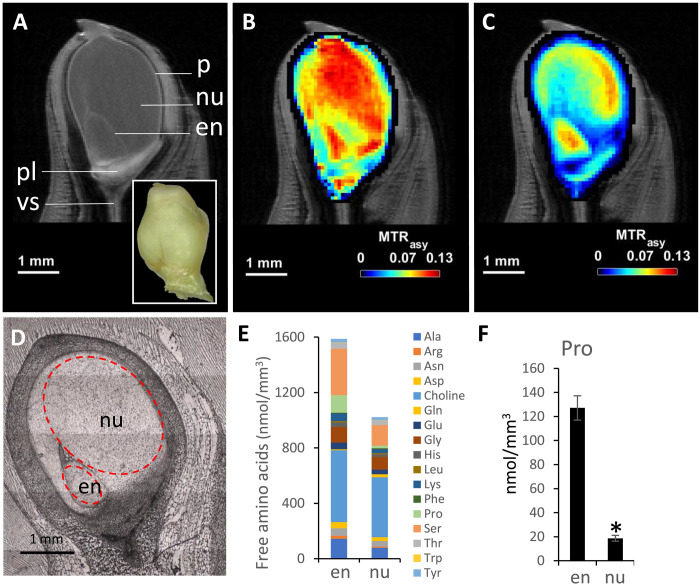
Noninvasive imaging of sugar and amino acid distribution by CEST and metabolite quantification by LC-MS in the early maize kernel. (A) MRI reference image visualizing the internal structure of the maize kernel (6 DAP). Photographic image of intact kernel on insert. (B and C) CEST image of sugars (B) and amino acids (C) in the living maize kernel. Image resolution, 40 μm. (D) Cryosection of the kernel used for microdissection of the tissues for detection of free amino acids; red dashed line indicates dissected regions. (E) Concentration of free amino acids measured in microdissected endosperm and nucellus using LC-MS. (F) Comparison of proline (Pro) level measured in microdissected endosperm and nucellus; *t* test, P* < 0.05; *n* = 3. cp, central placenta; ls, locule and seeds; nu, nucellus; pl, placental-hilar-funicular region.
